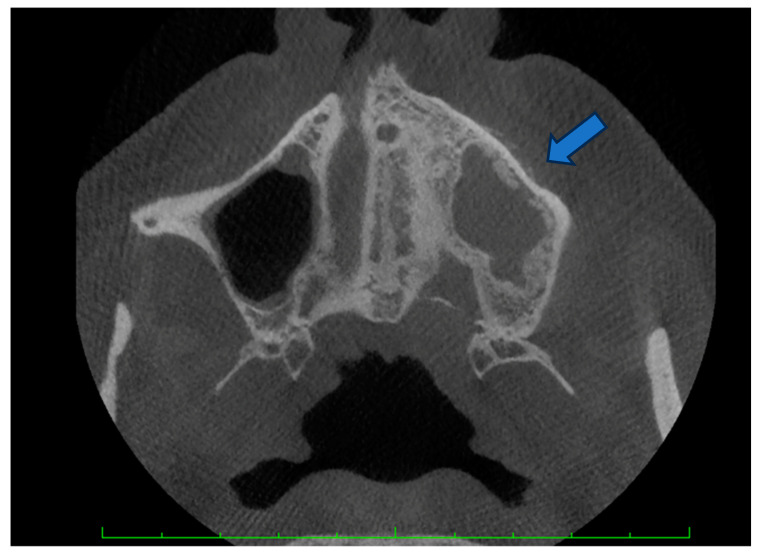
CBCT radiography: maxillary sinus involvement and cortical erosion. The arrow indicates the point at which bone resorption is appreciable.
